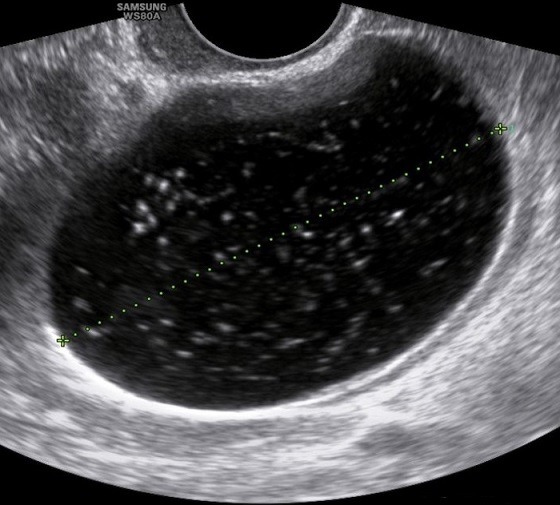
Transvaginal ultrasound image shows a low echogenic cystic mass with thin and smooth inner wall
